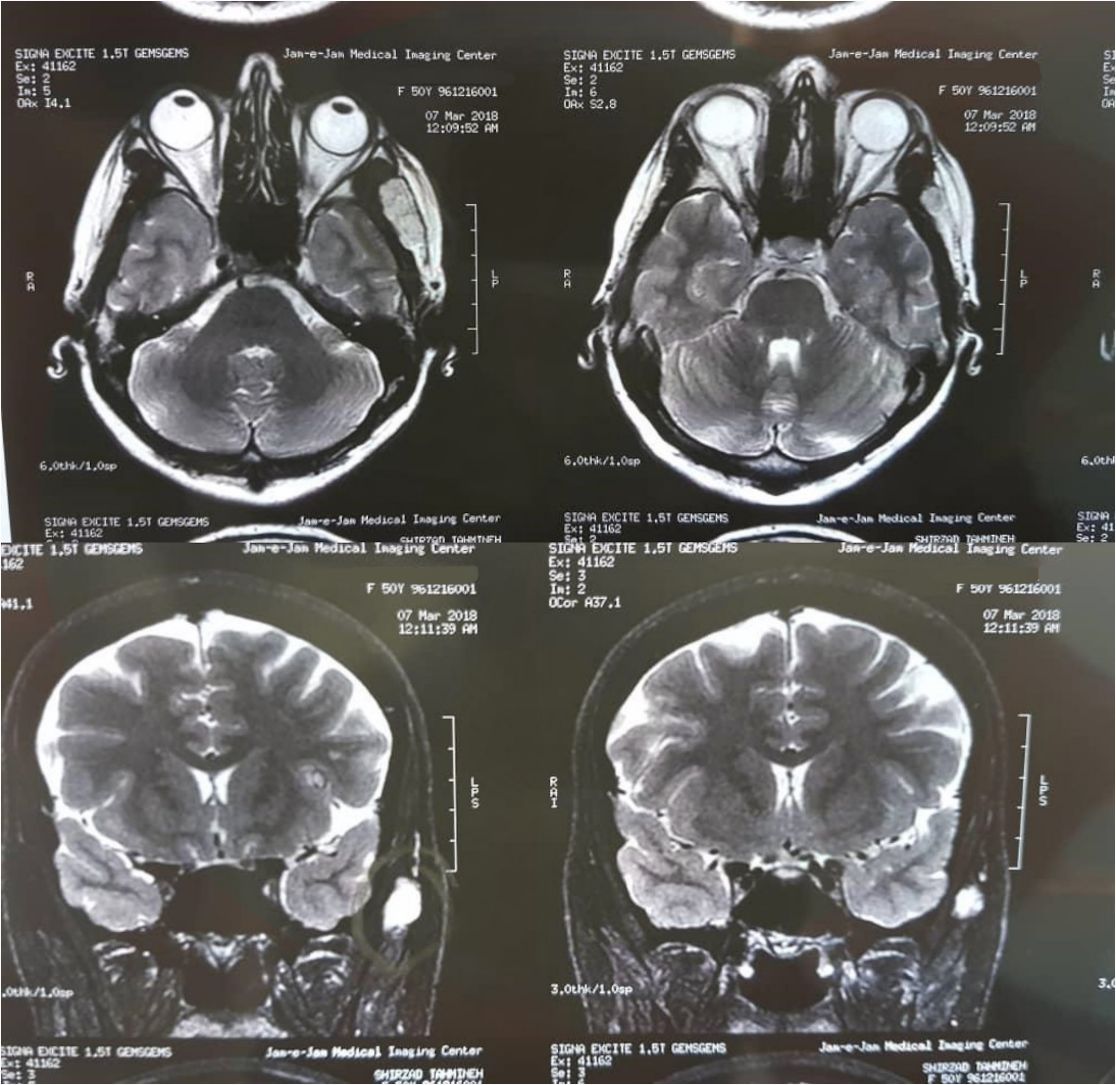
Axial (above) and coronal (below) T2 sequence magnetic resonance imaging showing a high‐intensity lesion within the left temporalis muscle.
